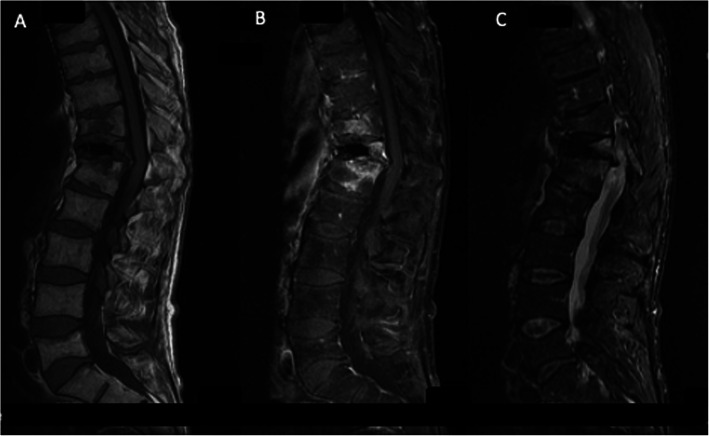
Spinal MRI, sagittal section 1 year later: A decrease in signal was observed. a T1-weighted; b T1-weighted with contrast agent; c STIR sequence
